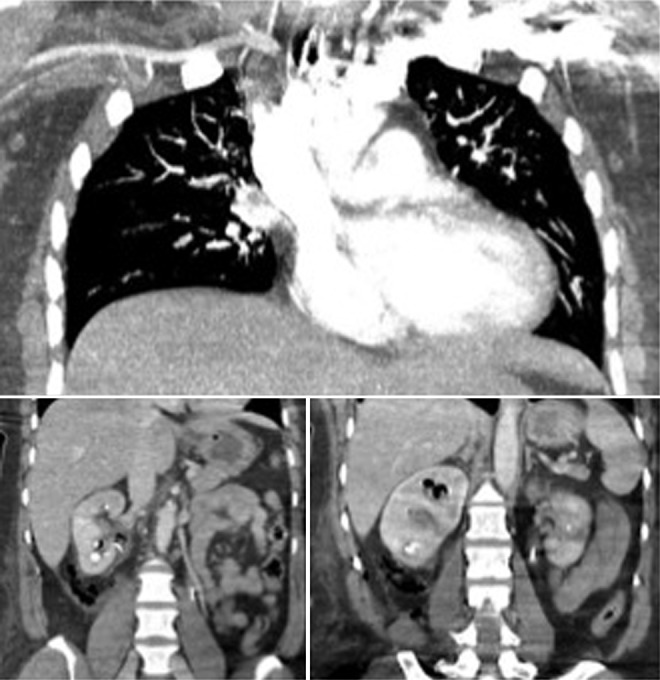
Intraoperative CT angiography.
